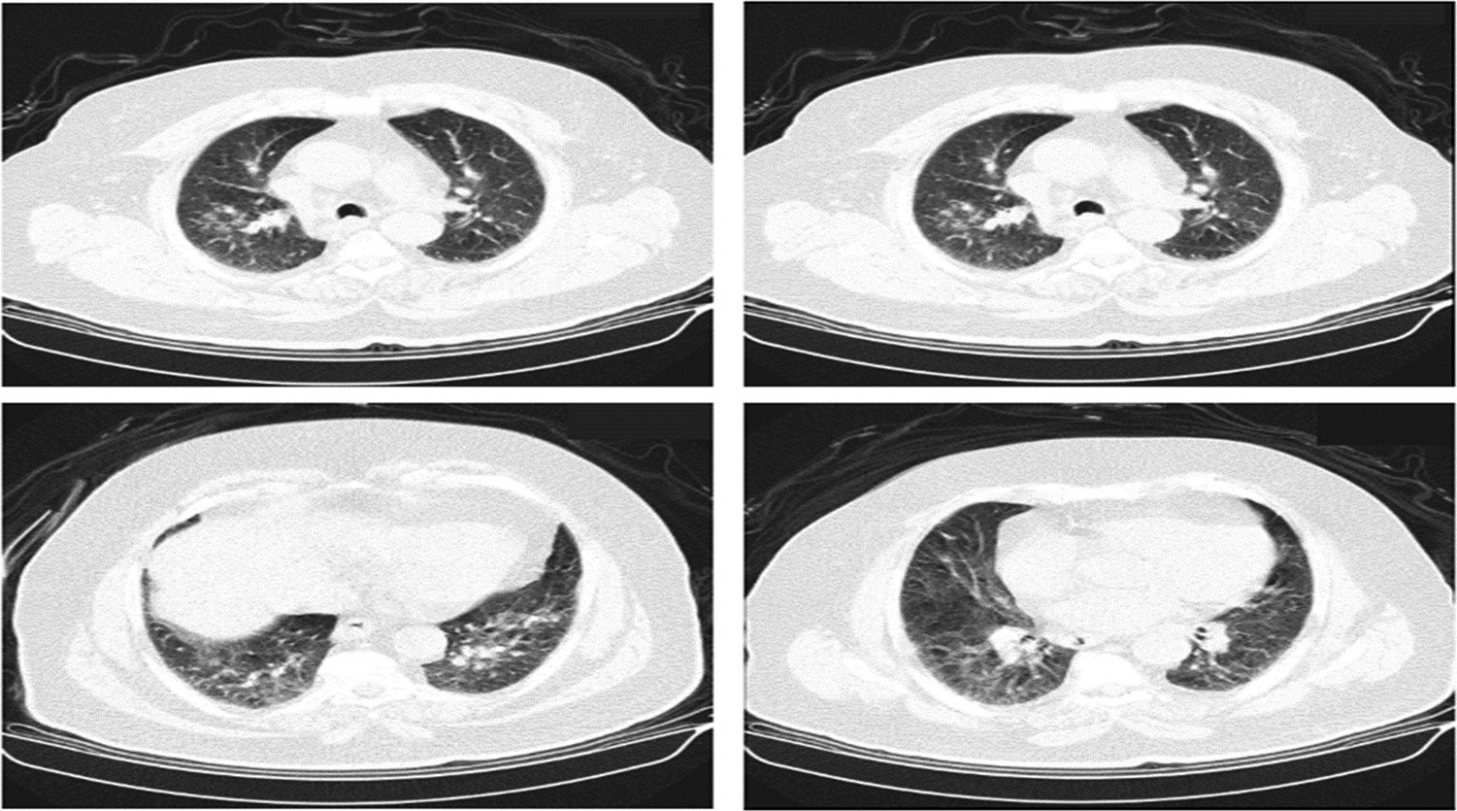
Computerized tomography (CT) on the lung. Multiple axial contiguous thin cuts were taken through the chest and have shown few right-side apical small ground-glass consolidation patches with bilateral mild subpleural lower lobar ground-glass haze more accentuated on the right side; in addition to mild to moderate cardiomegaly and right adrenal lesion with fat-rich adenoma.
